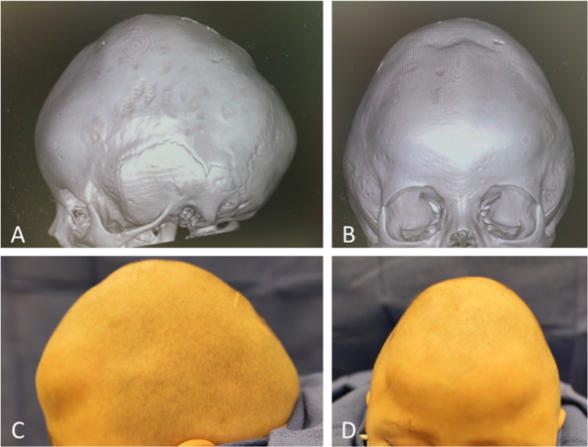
Pansynostosis and oxycephaly. Computed tomography images (A, B) and preoperative photos (C, D) for secondary corrective surgery showing pansynostosis and oxycephaly after inconsistent helmet therapy after endoscope-assisted strip craniectomy.
